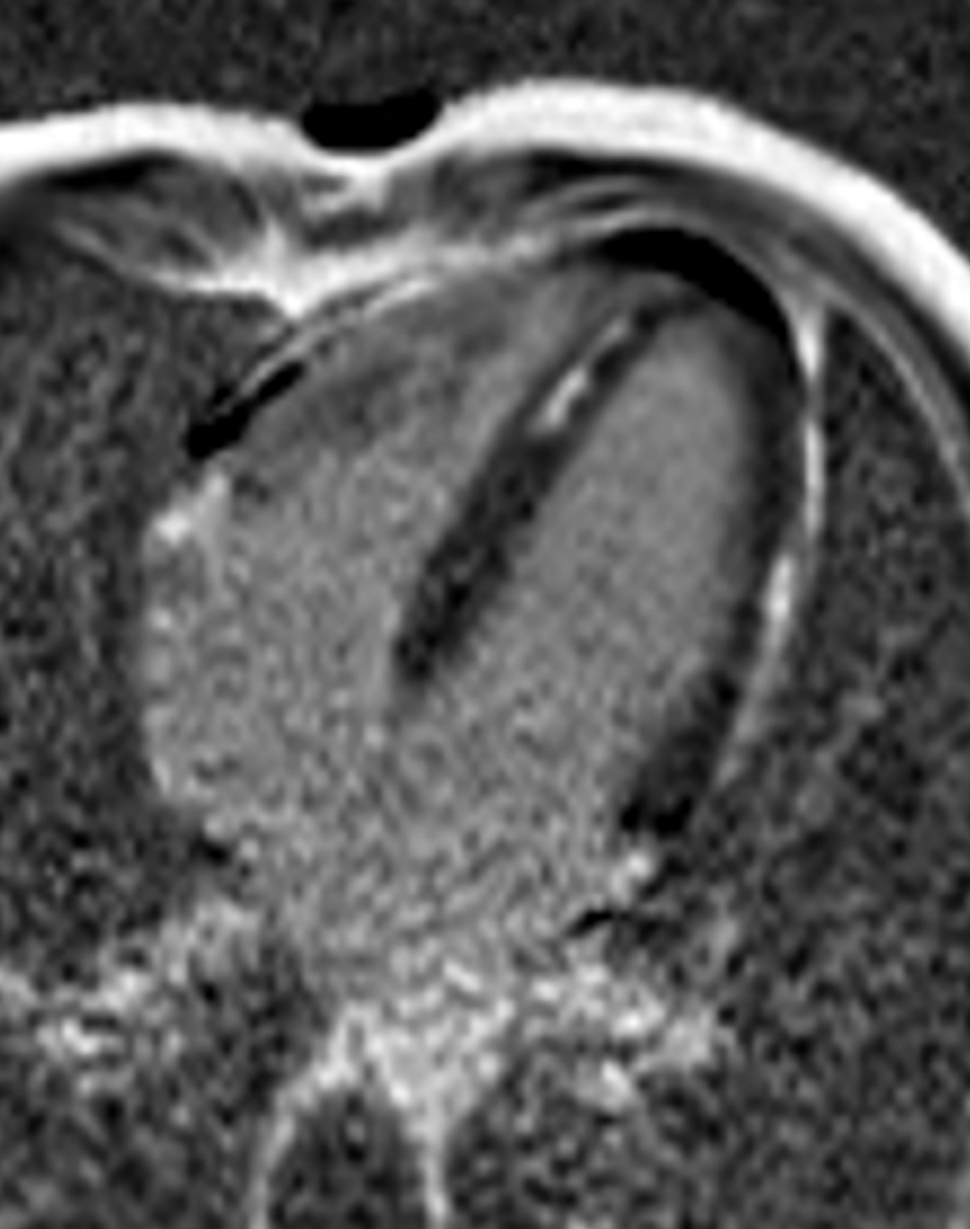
Cardiac MRI revealing myopericarditis
